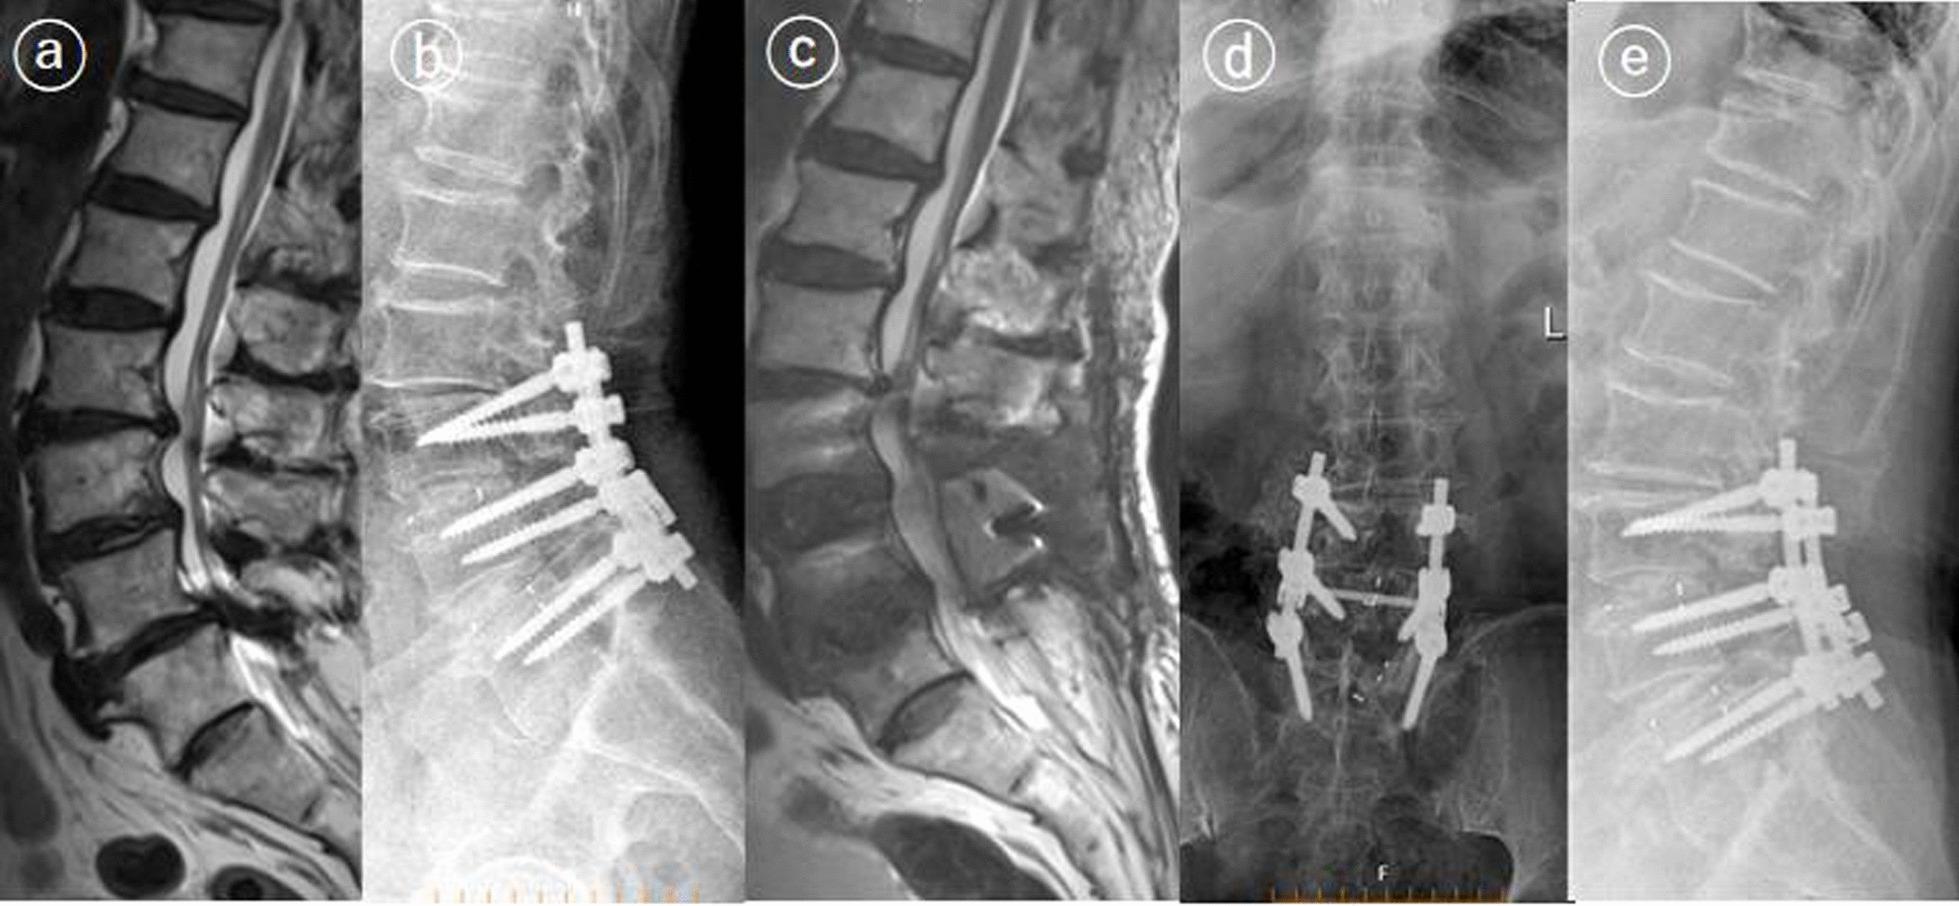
A 57-year-old patient underwent second surgery for persistent pain. The patient underwent decompression and posterior L3–L5 fusion surgery for lumbar spondylolisthesis and lumbar spinal stenosis (a–c). Preoperative leg pain was not adequately relieved after the first operation and lumbar spine MRI revealed residual stenosis at L2–L3 and L3–L4 (c). After adequate decompression surgery, the patient’s pain was relieved (d, e)
